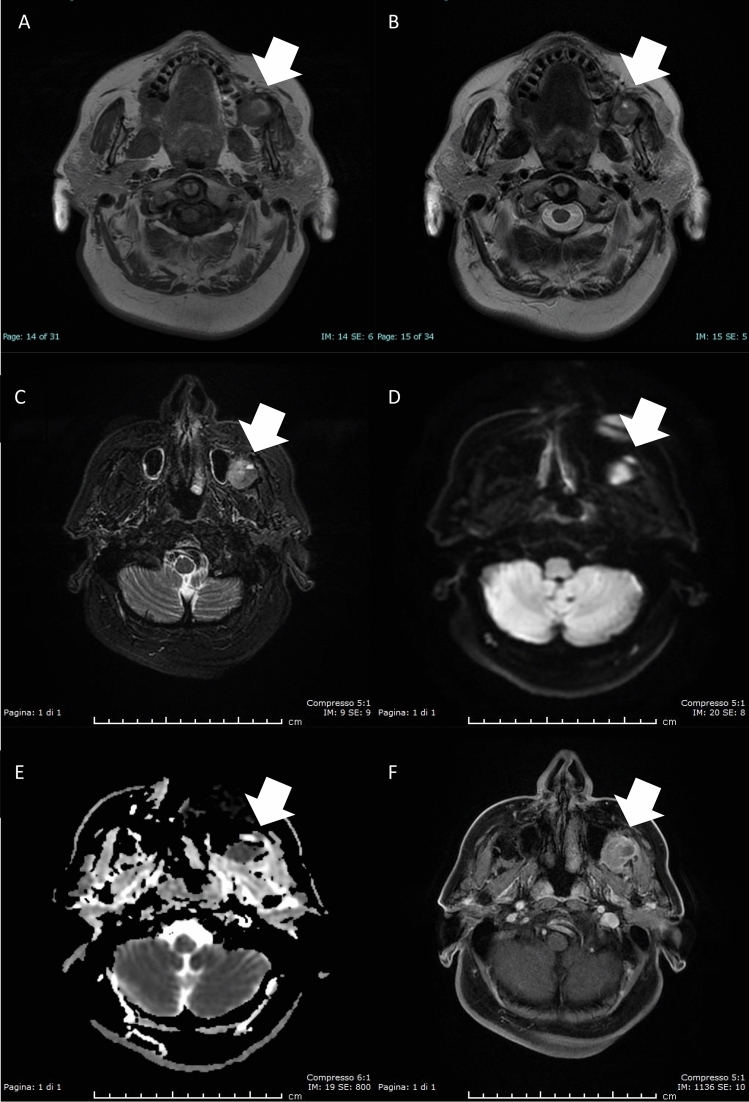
Magnetic resonance imaging revealed a solid mass located in the left buccal space. a The lesion (arrow) showed a focal component with high signal intensity on axial T1-weighted imaging and b low signal intensity on T2-weighted imaging and c on fat-saturated T2 STIR sequences. d Diffusion-weighted imaging revealed a restricted diffusion change with e low apparent diffusion coefficient of 0.9. f After intravenous contrast administration, the lesion showed increased signal intensity on T1-weighted imaging
